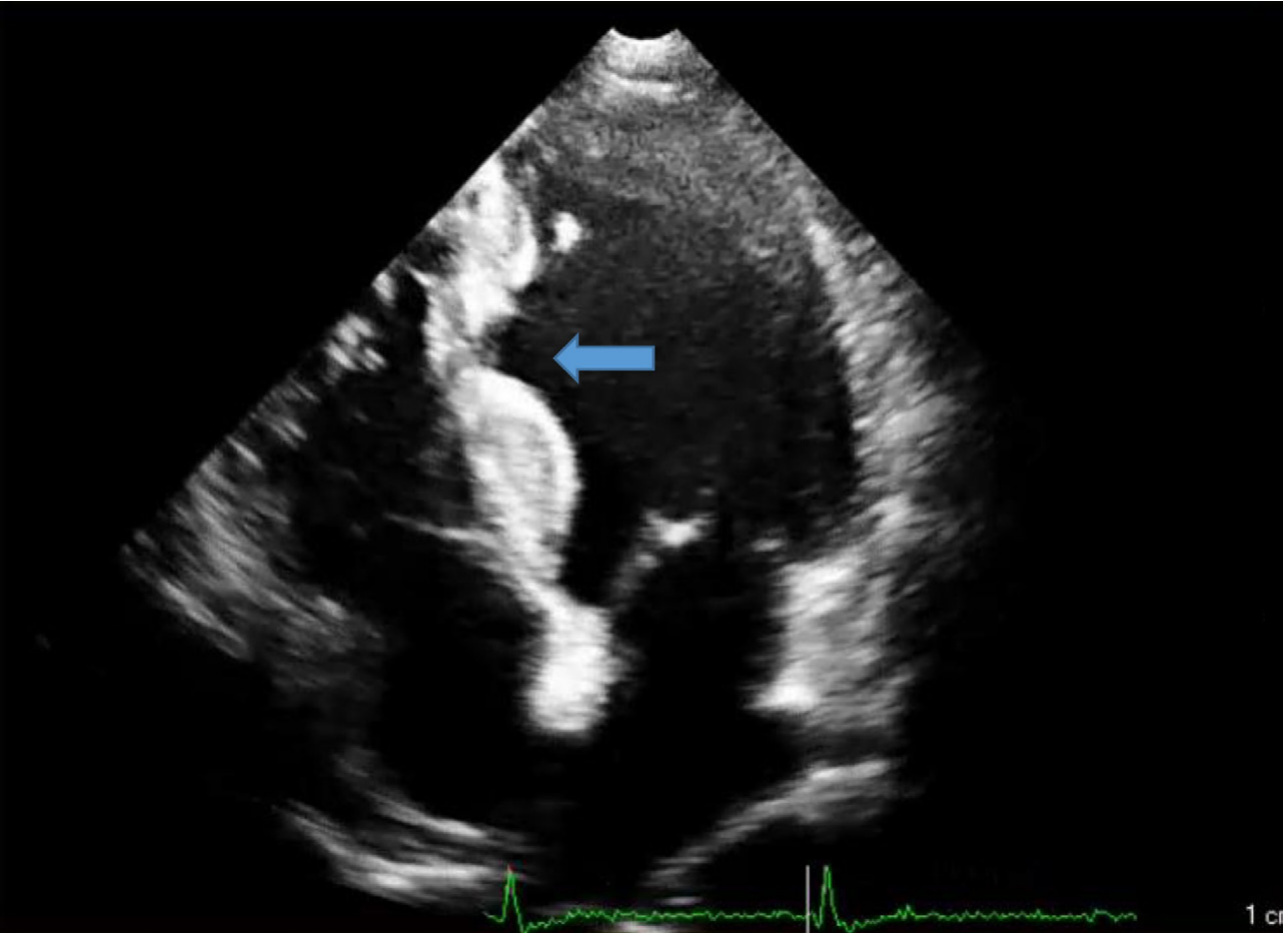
Four-chamber view of two-dimensional transthoracic echocardiography, showing a diverticulum in the mid inferoseptal wall (arrow)
